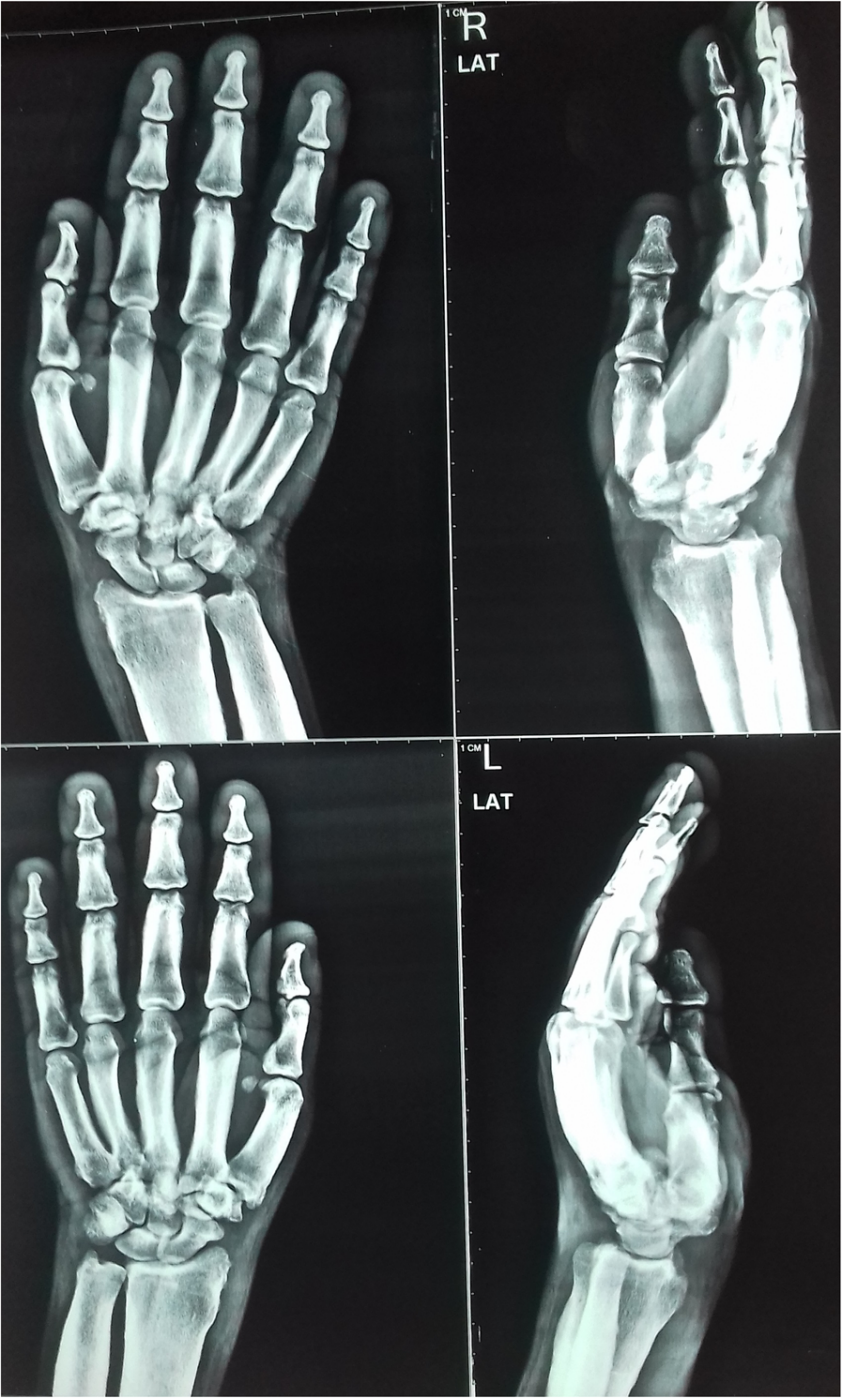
Radiograph of bilateral hands showing soft tissue tumefaction, hyperostosis of metacarpal, and proximal phalanges
